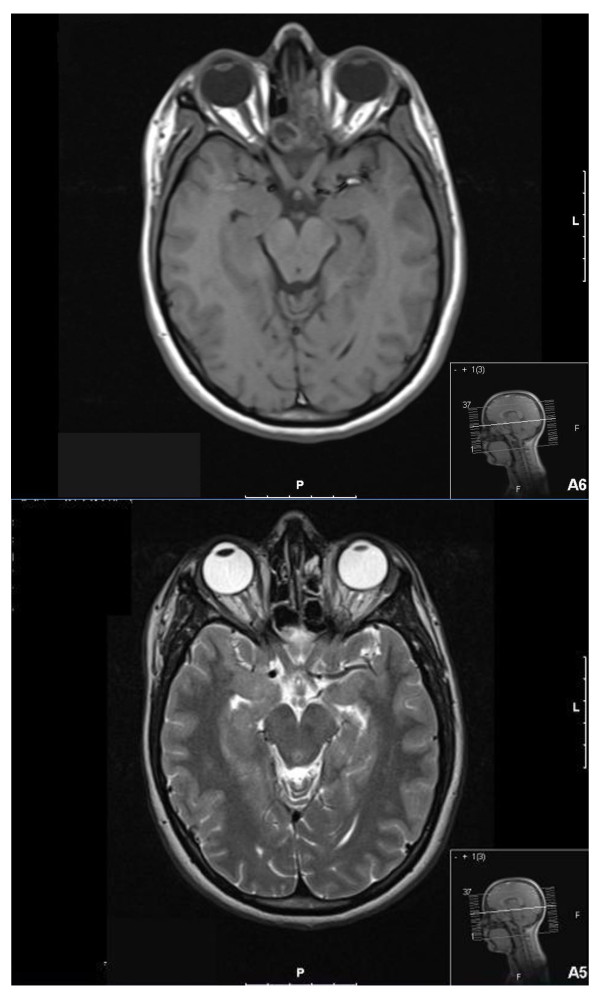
Magnetic resonance images of sinuses demonstrate a recurrence of the fungal infection. Axial T1-weighted and T2-weighted images show opacification of the sphenoid sinus on the left with signal characteristics of fungal infection. The infection extends into the left posterior and anterior ethmoidal air cells.
